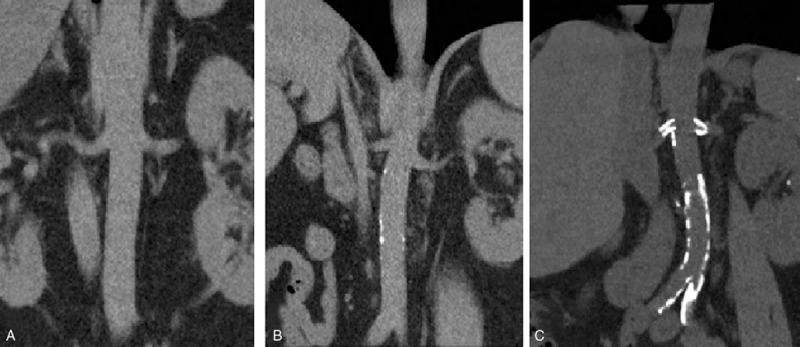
Computed tomography-angiography reconstruction of the renal arteries and part of the aorta of 3 patients with respectively a low (A), intermediate (B), or high calcium score (C). The ambulatory blood pressure measurements response for these patients 6 months post-RDN was respectively: −17 mmHg (A), −25 mmHg (B), and −13 mmHg (C).
